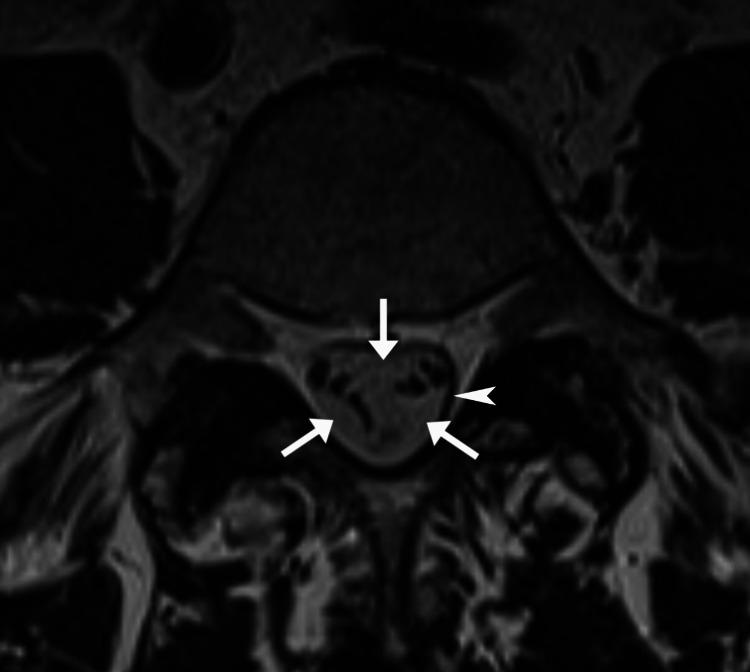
Axial lumbar MRI A T2-weighted section at the level of the L5 vertebra shows crowding of nerve roots centrally with denticulate ligaments and midline dorsal septum separating the subdural collections into an anterior collection and two posterolateral collections (arrows). The hematoma also follows the rounded contour of the thecal sac (arrowhead), a feature that helps distinguish this condition from an epidural hematoma. MRI: magnetic resonance imaging
